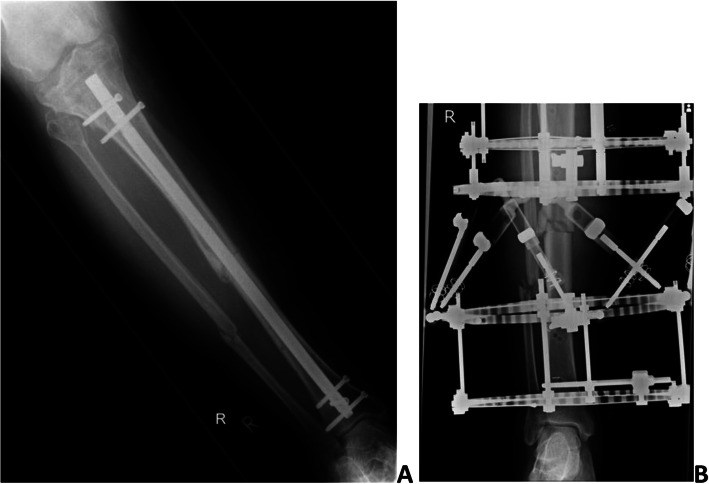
a Radiograph showing segmental tibial fracture fixation with IMN. b Radiograph showing CFEF fixation
